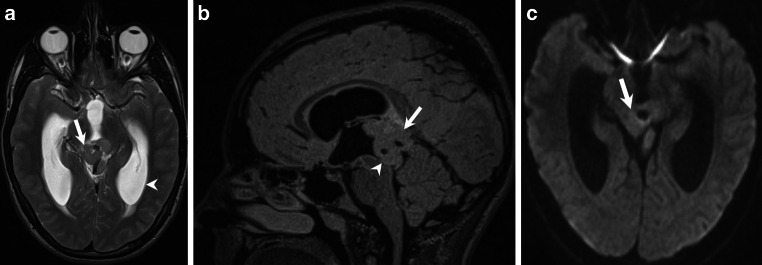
Axial T2-weighted images (a) show an isointense lesion (*arrow*) located in the pineal region which lead to subsequent triventricular dilatation (*arrowhead*). On sagittal FLAIR (fluid-attenuated inversion recovery) images (b) the lesion (*arrow*) compresses the aqueduct (*arrowhead*). On axial diffusion-weighted images (c) the lesion (*arrow*) does not show any signs of restricted diffusion
